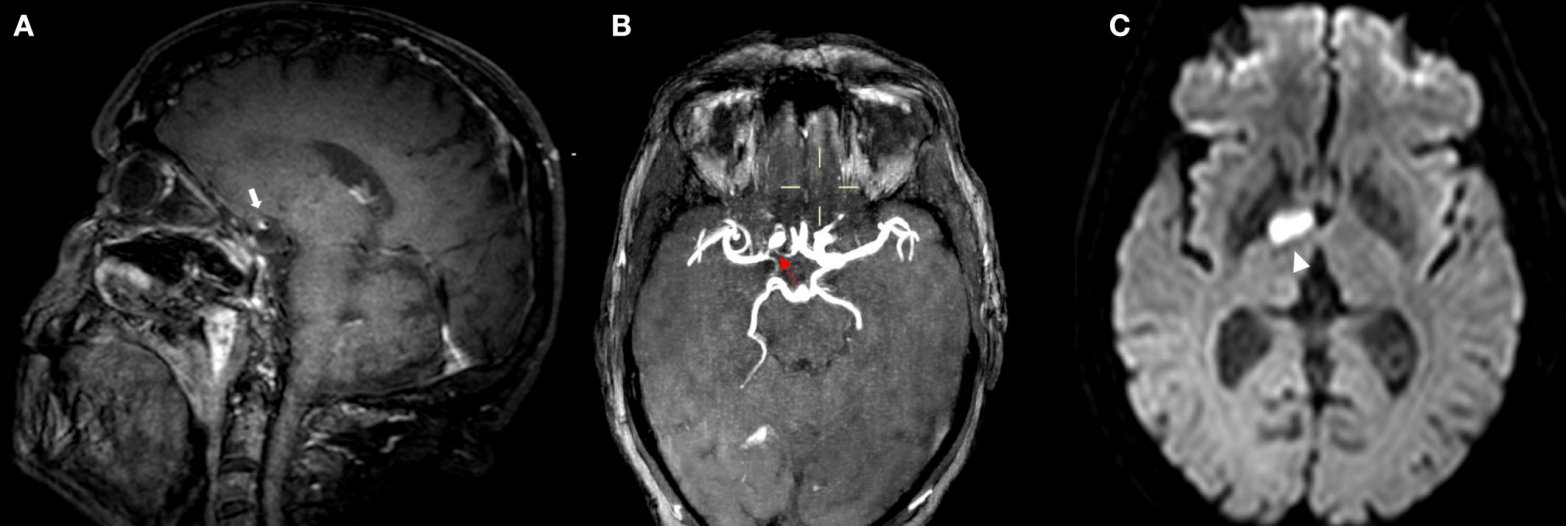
MRI of a patient presenting with ICAD. IVW-MRI showing eccentric contrast enhancement in the junction between right internal carotid and middle cerebral [(A) white arrow] which correspond to a stenosis in the same vessel segment in the time of flight-MRA sequence [(B) red arrow]. Patient presented with an acute infarction in the capsule-lenticular area [(C) arrowhead].
